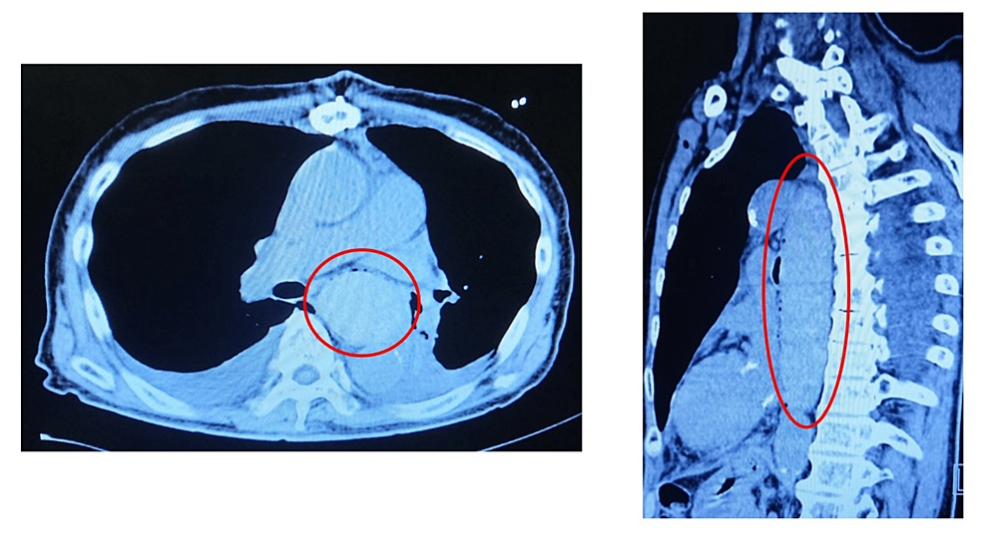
Chest CT images, axial (left) and sagittal (right) views, taken on postoperative day 3. The images show a giant esophageal submucosal hematoma (45×50×210 mm). CT: computed tomography
